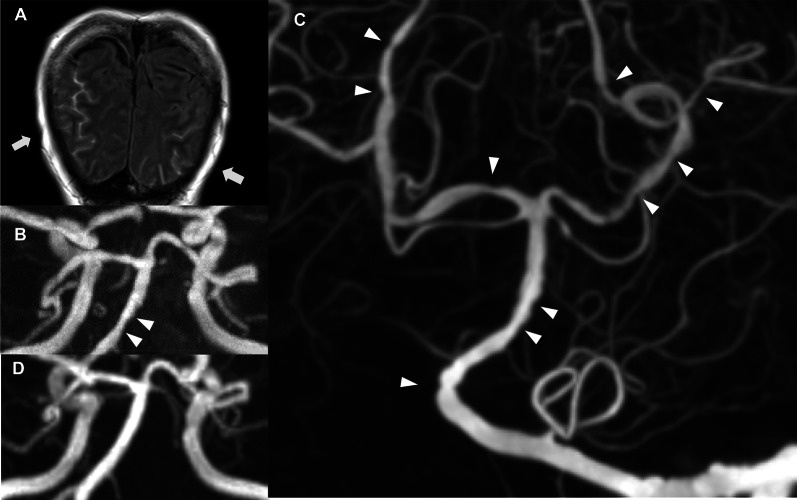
MRI images of the head. A Fluid-attenuated inversion recovery image on day 24 of hospitalization. The high-signal area along the sulcus suggested SAH (white arrows). B MRA image on day 24 of hospitalization, showing wall irregularity in the basilar artery (white arrowheads). C Cerebral angiography on day 26 of hospitalization. No signs of cerebral aneurysm were observed. Wall irregularities are visible in the left vertebral artery, basilar artery, and bilateral posterior cerebral arteries (white arrowheads). D MRA image 5 months after discharge. The irregularities in the wall of the basilar artery had completely disappeared. *MRA* magnetic resonance angiography, *MRI* magnetic resonance imaging, *SAH* subarachnoid hemorrhage
